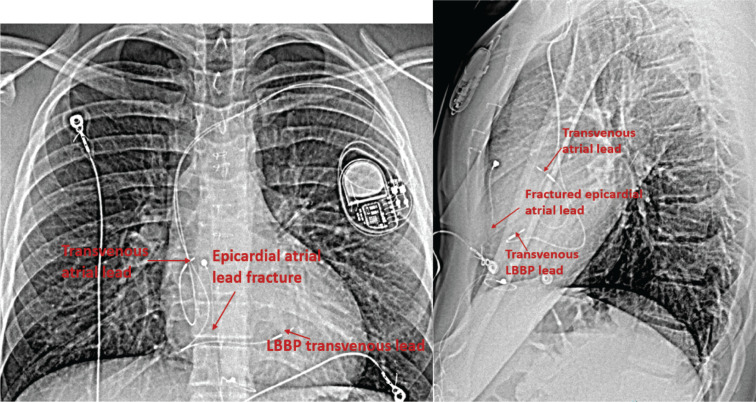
The postero-anterior and lateral chest X-ray post-transvenous left bundle branch system and atrial epicardial pacing lead fracture.
